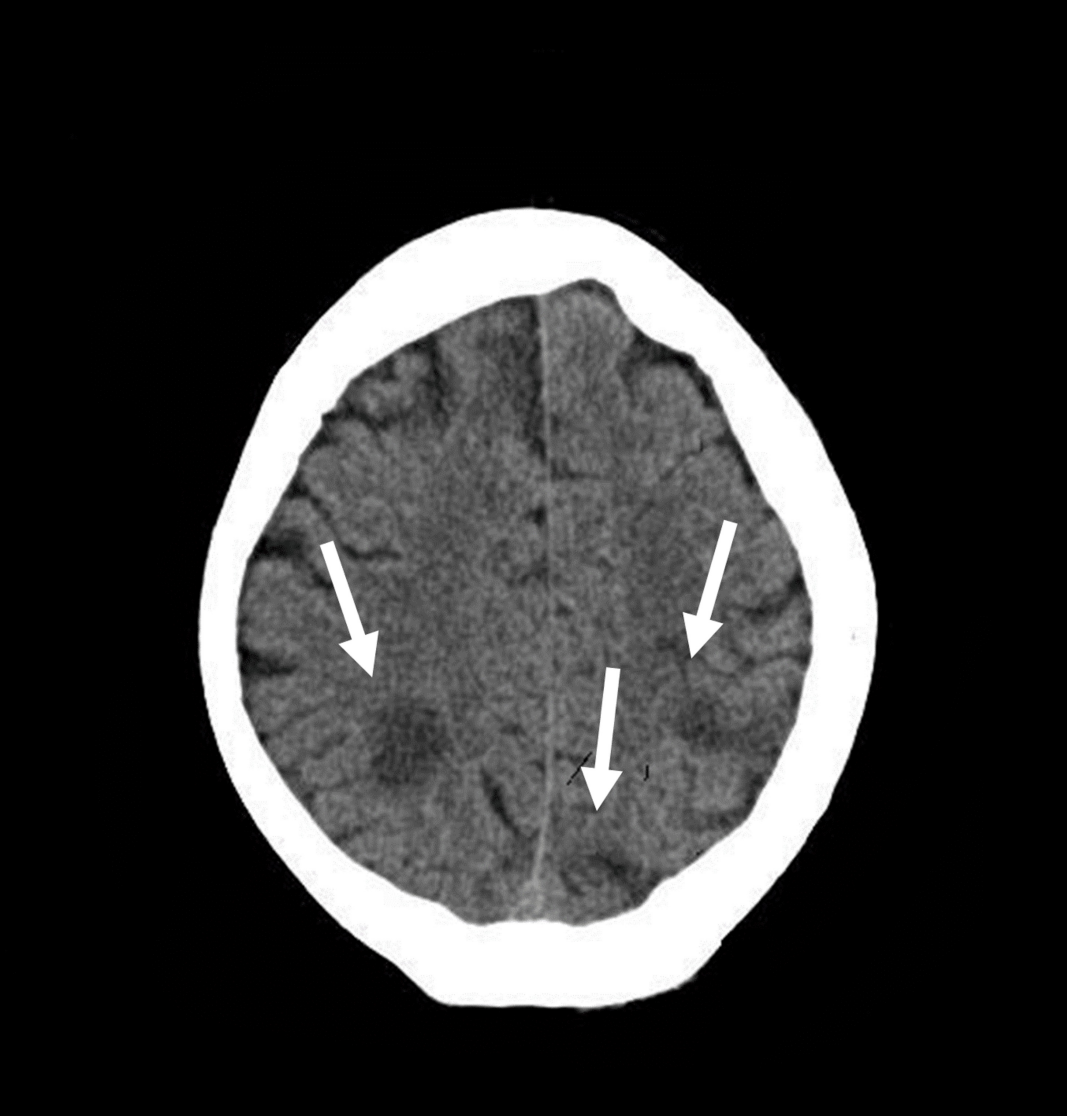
CT head with contrast showing new bilateral parietal, left parieto-occipital and right frontal lobes infarcts. Axial non-contrast CT image demonstrating new areas of hypodensity involving the bilateral parietal, left parieto-occipital, and right frontal lobes, consistent with recent multifocal ischaemic infarcts. (Image obtained from the hospital PACS system with patient consent.) Arrows indicate multiple areas of hypodensity, indicating acute infarcts.
